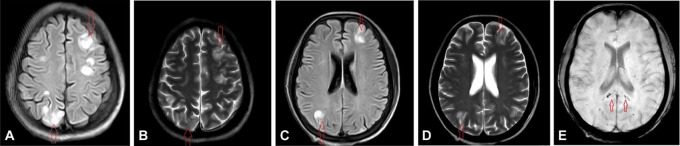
A) MRI Brain-FLAIR sequences showing hyperintensity in the left frontal and right parietal region, B) MRI Brain-T2 hyperintensity in left frontal and right parietal region, C) MRI Brain-FLAIR sequence showing hyperintensity in the left frontal and right parietal region at the level of lateral ventricles, D) MRI Brain-T2 hyperintensity in left frontal and right parietal region, E) MRI Brain-SWI sequence showing petechial hemorrhages in splenium of corpus callosum.
